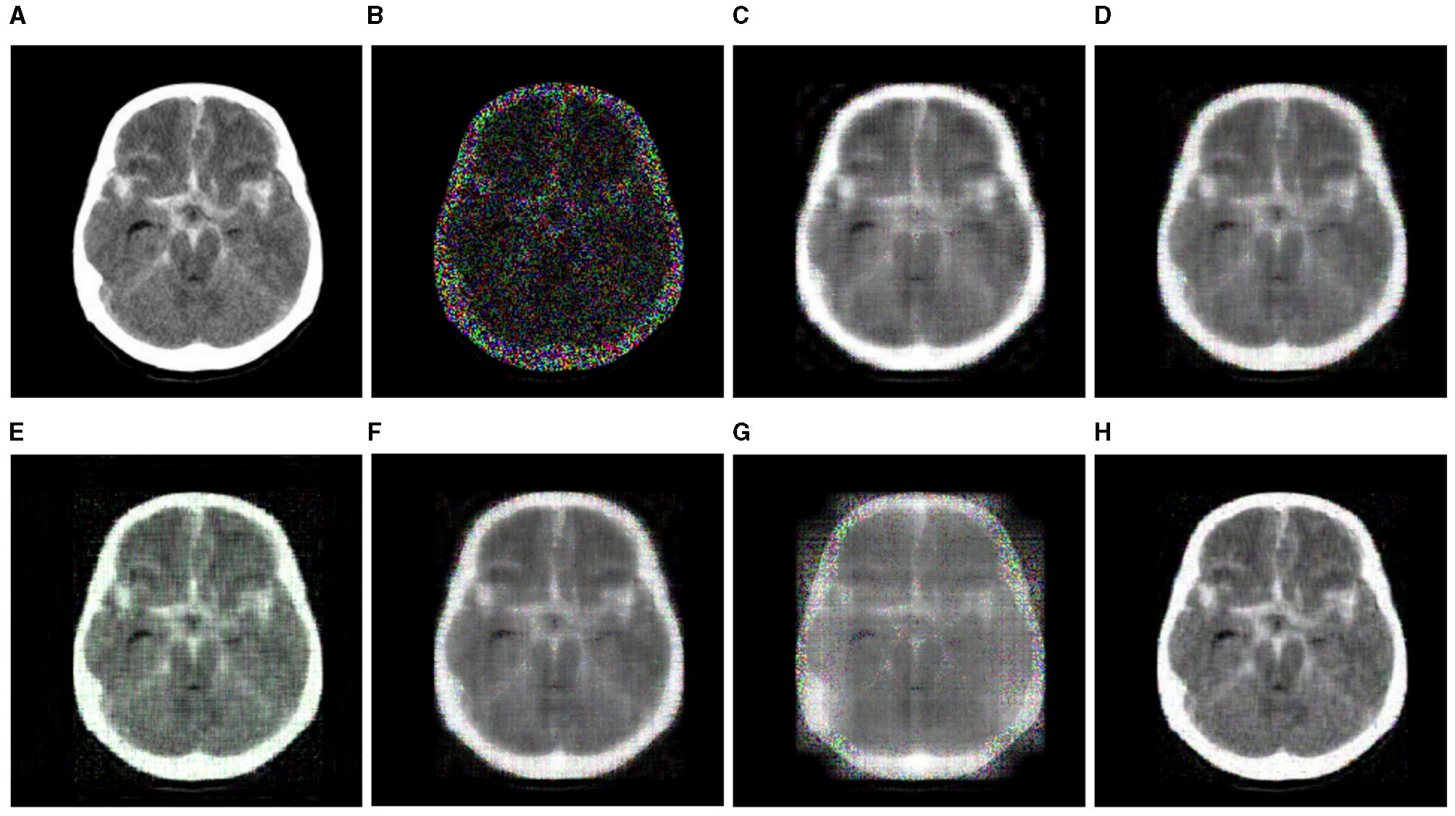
Completion visualization results of brain MRI slice data at 70% MR (best viewed zoomed in). (A) Original (adapted from https://www.kaggle.com/datasets/tourist55/alzheimers-dataset-4-class-of-images). (B) Observation. (C) CP-WOPT. (D) FaLRTC. (E) TT-SGD. (F) gHOI. (G) CTNM. (H) CTNM-QR.
